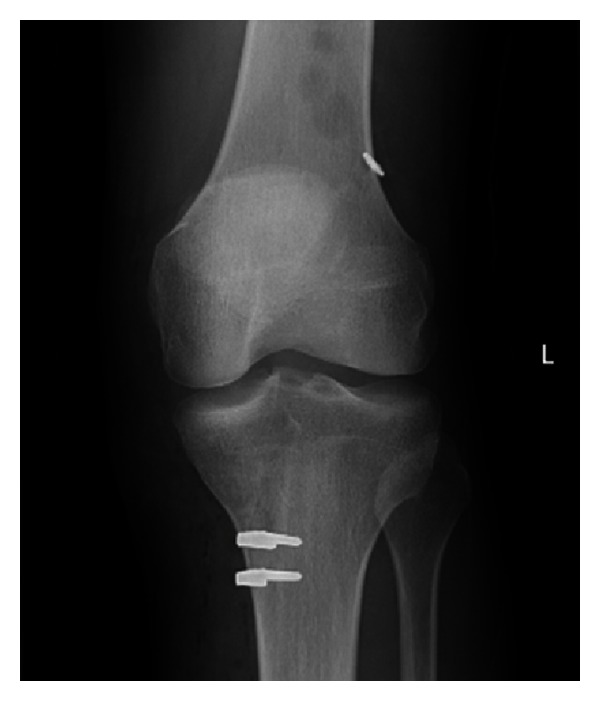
X-ray of the septic ACL reconstructed knee.
